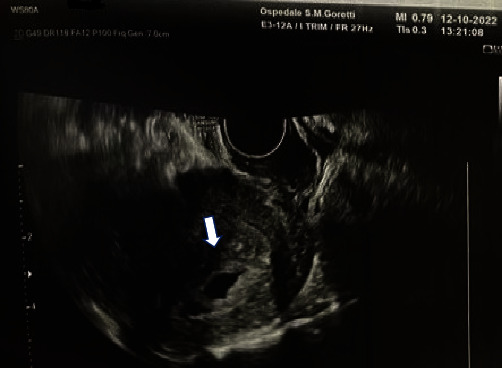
Pelvic TV ultrasound scan at 5 weeks of amenorrhea. The image shows an anechoic intrauterine scan compatible with a hemerion-free gestational chamber without cardiac activity and diffuse trophoblast characterized by an irregular profile. Both adnexa are normal. Reassessment is required after a week.
